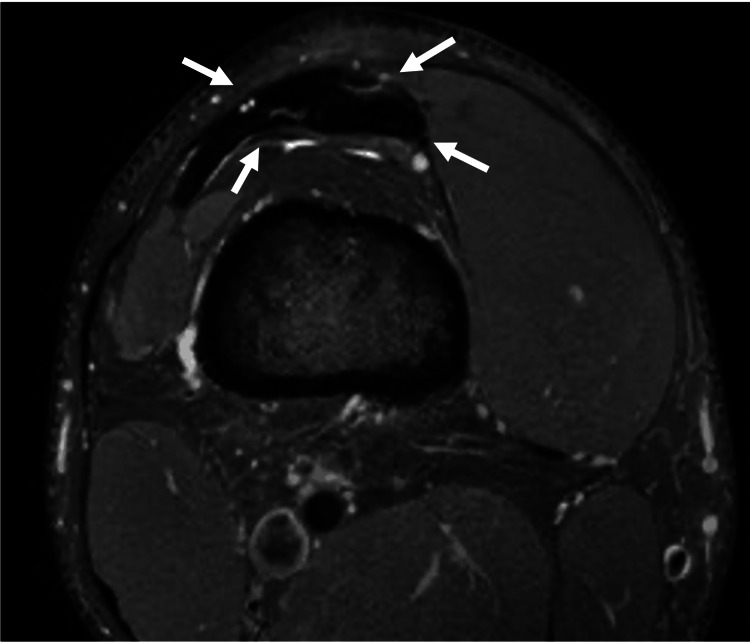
Mild quadriceps tendinosis in a 21-year-old man. Axial proton density fat-suppressed MRI of the knee showing mild distal quadriceps tendinopathy. Arrows: Quadriceps tendon.
